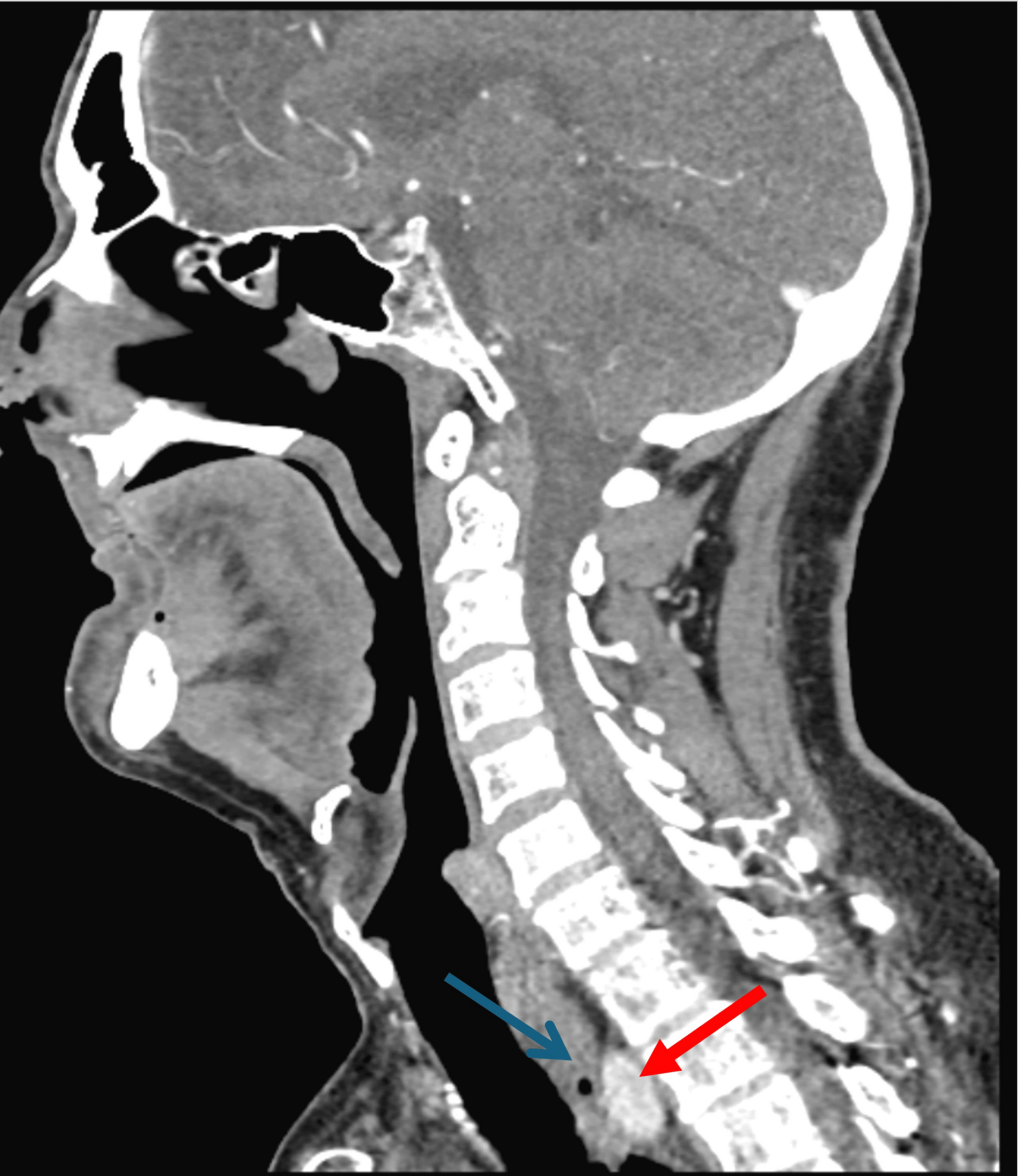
Sagittal view of the CT scan of the head and neck with the aberrant right subclavian artery (red arrow) overriding the esophagus (blue arrow).
